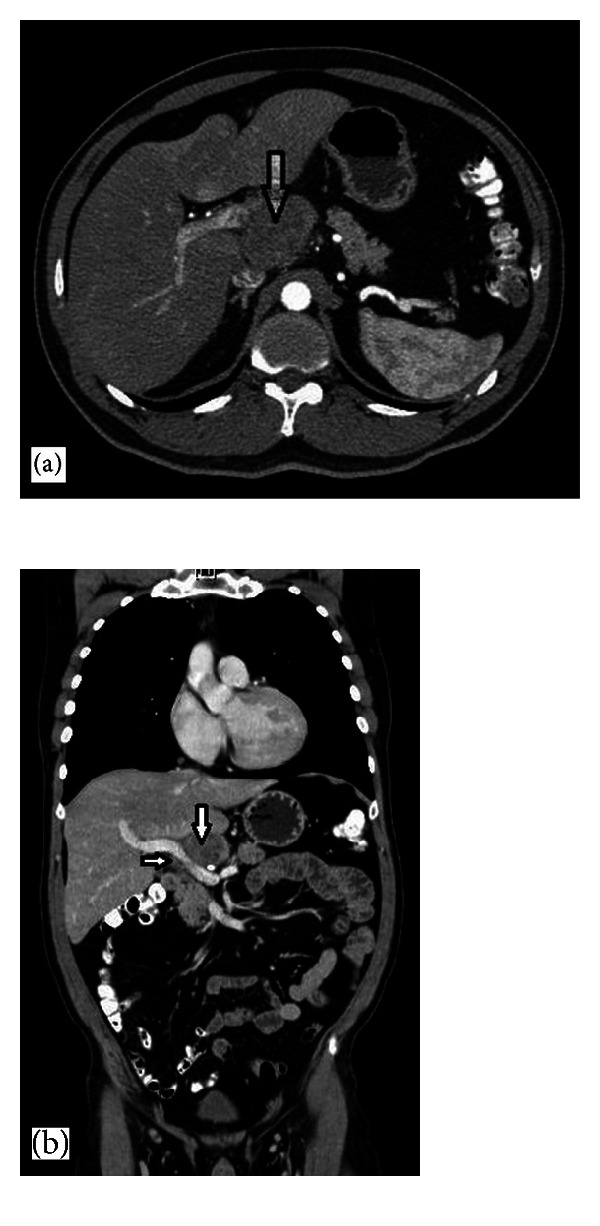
Transverse (a) and coronal (b) images of contrast enhanced CT showing upper abdominal mass located at liver hilum, containing heterogenous lymphadenopathy (arrows).
